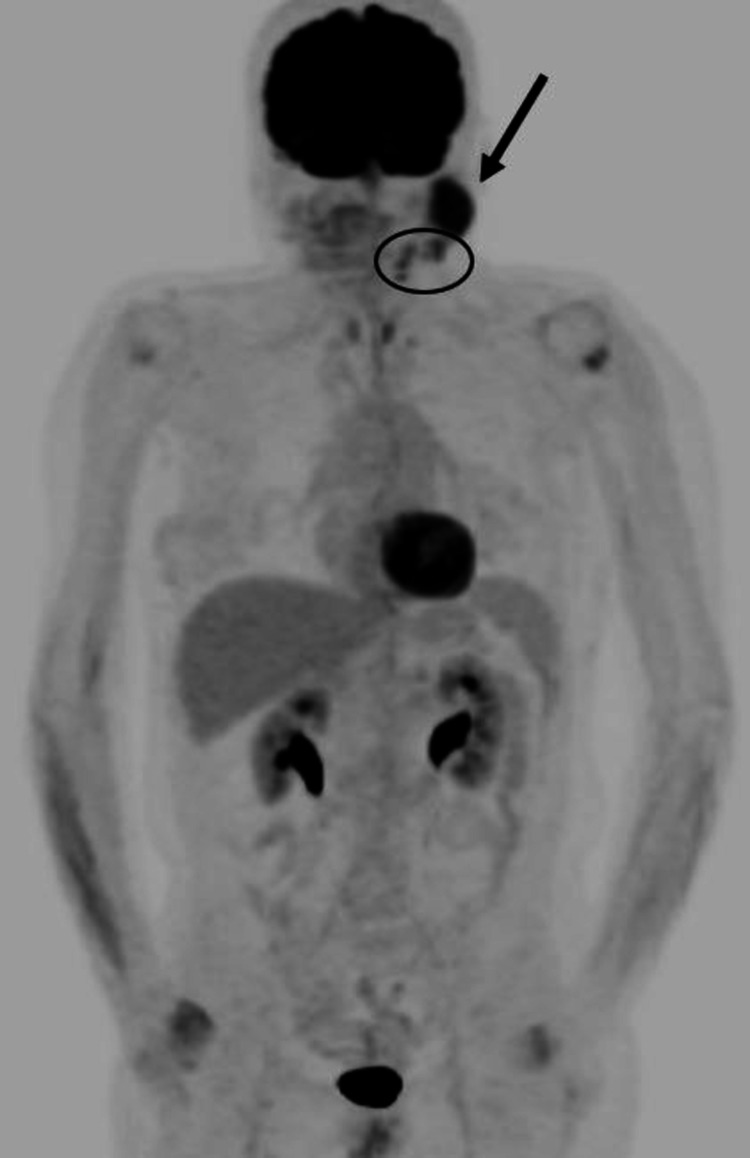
FDG-18 PET MIP image showing intense radiotracer uptake in left parotid space mass (black arrow). The additional sites of radiotracer uptake in left level II cervical lymph nodes (black circle) consistent with nodal involvement. The patient information at the time of injection: blood glucose - 84 mg/dL, weight - 122 lbs, BMI - 22 kg/m^2^, and time to injection - 64 minutes. FDG-18: fludeoxyglucose F-18; PET: positron emission tomography; MIP: maximum intensity projection
